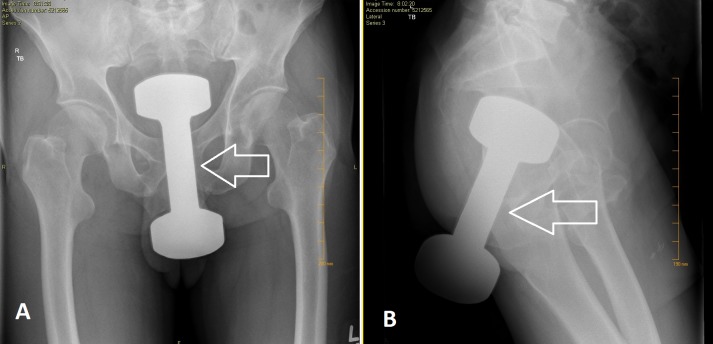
Rectal dumbbell (arrow) on abdominal plain films (A) antero-posterior view and (B) lateral view.
